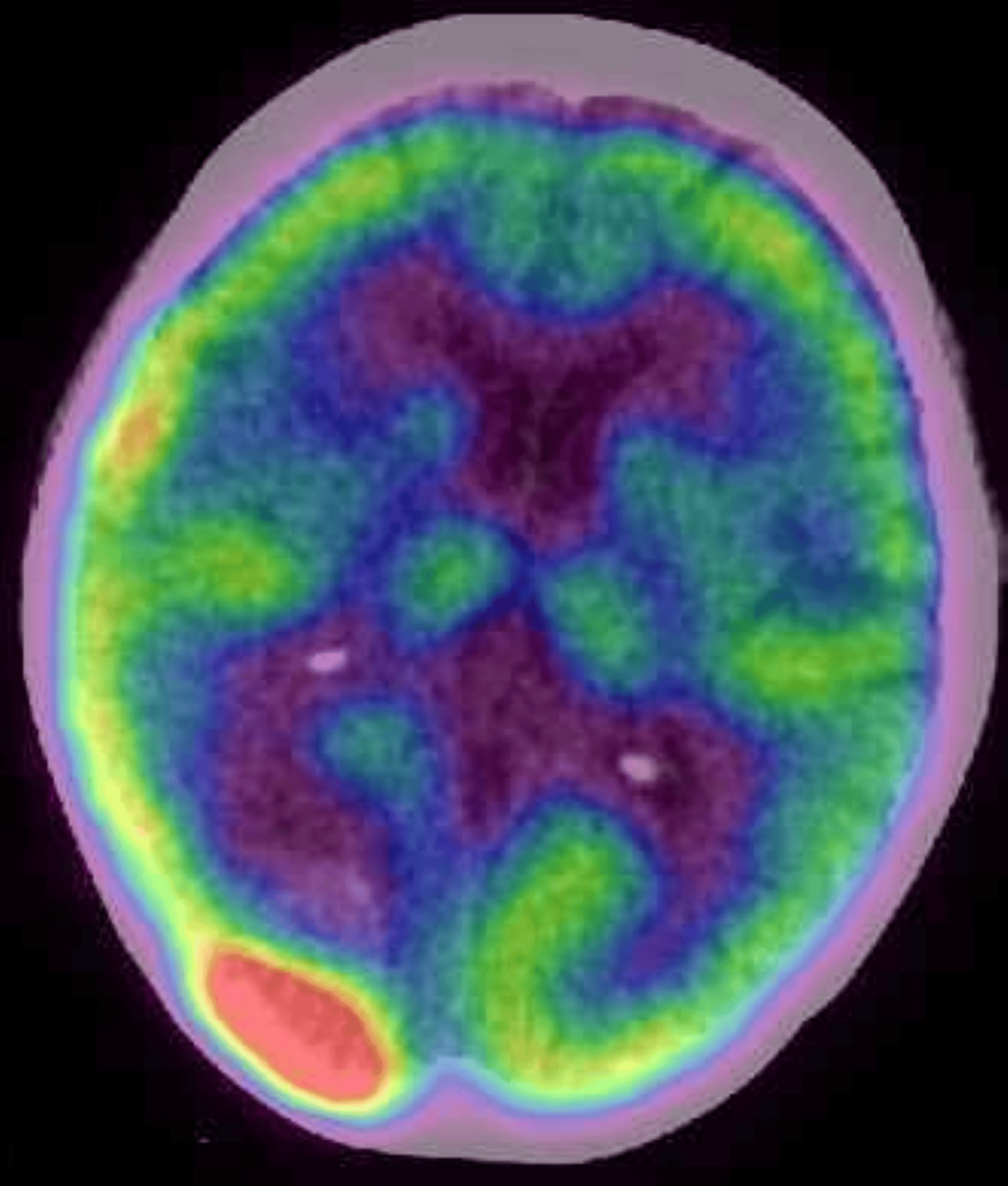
PET-CT image at relapse showing a mass with focal abnormal accumulation of fluorodeoxyglucose in the right occipital lobe PET, positron emission tomography; CT: computed tomography
